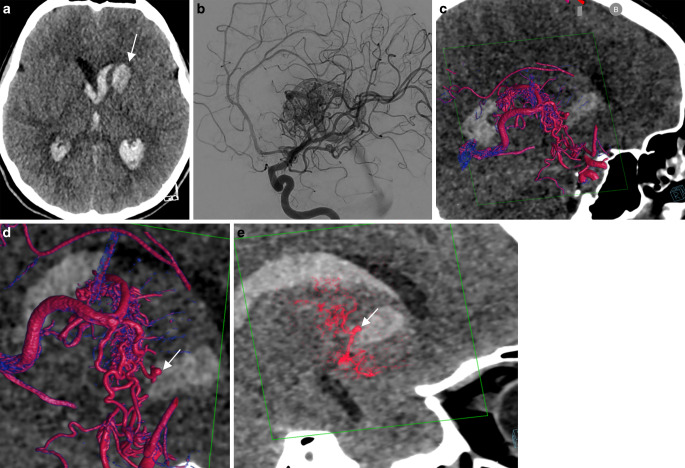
Ruptured periventricular arteriovenous malformation (AVM) with an intranidal aneurysm. a The non-enhanced computed tomography (CT) demonstrates a hemorrhage in the left caudate nucleus (*arrow*) with intraventricular rupture. b The digital subtraction angiography (DSA) shows a left basal ganglia nidus supplied by the anterior choroidal artery, draining via a single vein into the deep venous system. c,d Fusion of the non-enhanced baseline CT scan and three-dimensional rotational angiography (3DRA) shows a small intranidal aneurysm centered in the left caudate nucleus as the bleeding source. e Fusion of the baseline CT and superselective 3DRA with the microcatheter placed in the feeding artery supplying the aneurysm and the anterior part of the nidus
